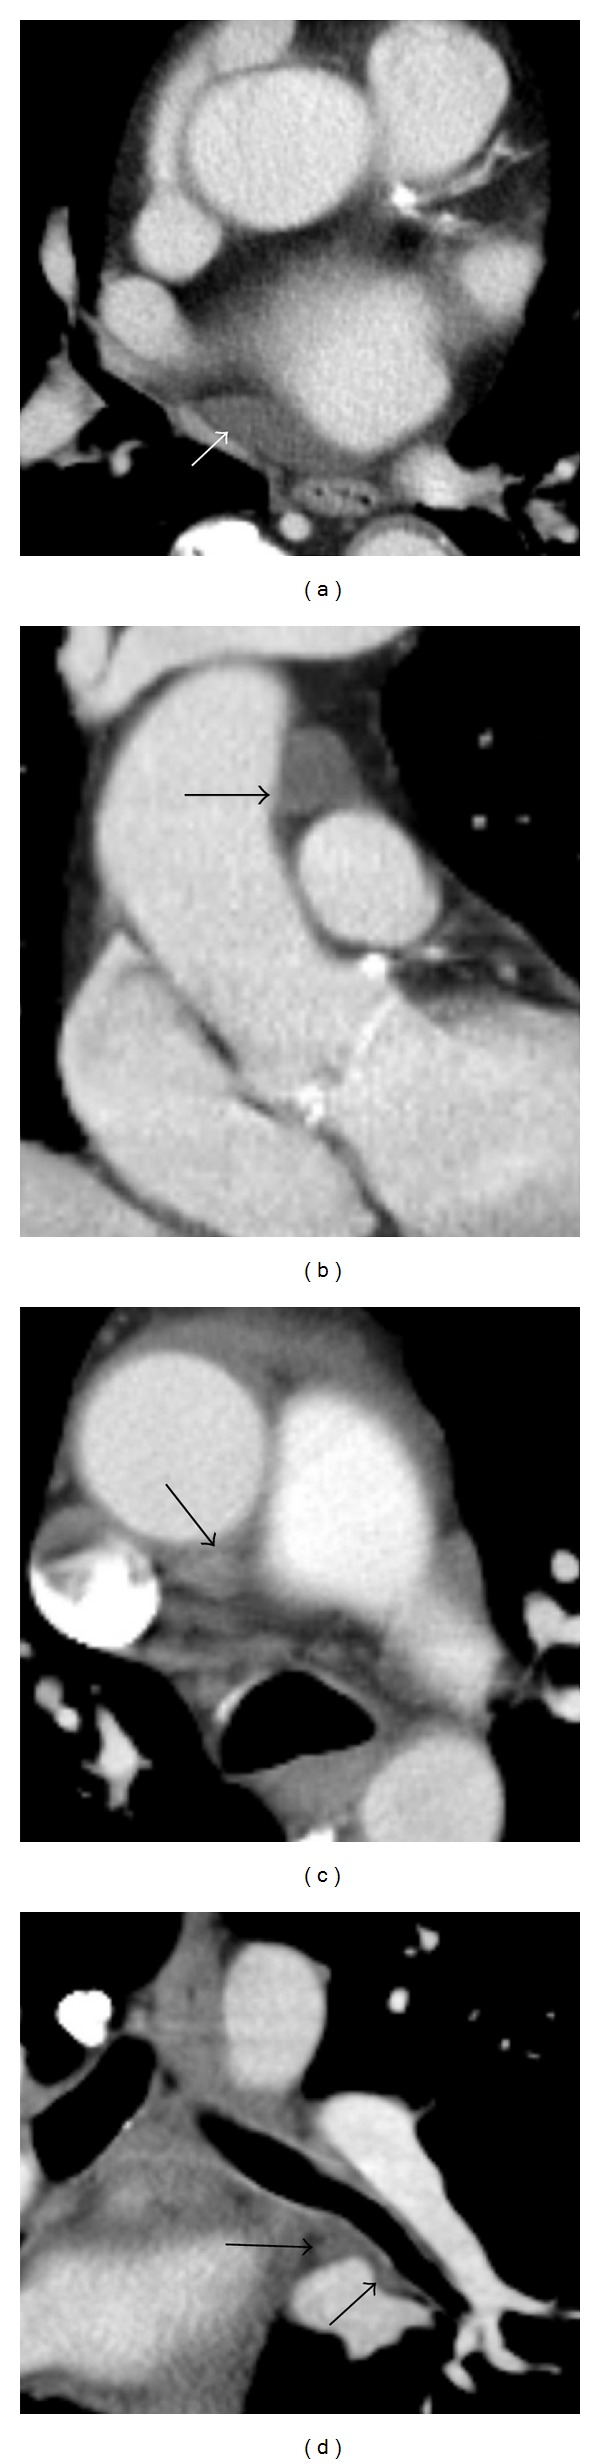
Normal pericardial recesses may be confused with pathology. (a) Contrast-enhanced CT (CECT) demonstrates fluid in the oblique sinus (white arrow). (b, c) Fluid in the superior aortic recess (black arrows). This may appear dense on CT due to contrast blooming and maybe mistaken for soft tissue. (d) Fluid in the left inferior pulmonary venous recess (black arrows).
